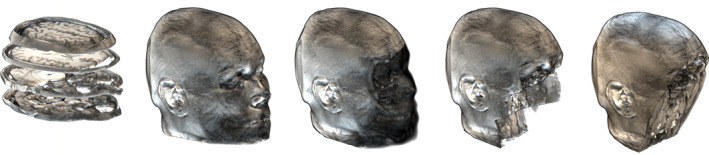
The typical structural MRI of the brain is made up of a series of 2D slices (left) from which it is easy to reconstruct a face. Pseudonymization procedures (from the middle to right) go from blurring/masking the face to zero‐out an entire part of the image, increasing anonymity but decreasing usage and sometimes damaging the frontal part of the brain. (This image was made from the MRI of one of the authors, CP, visualized with MRICRoGL, masked using mask_face (https://nrg.wustl.edu/software/face‐masking/usage/), mri_deface from the freesurfer suite (https://surfer.nmr.mgh.harvard.edu/fswiki/mri_deface) and SPM12 (https://www.fil.ion.ucl.ac.uk/spm/software/spm12/) — (https://doi.org/10.7488/ds/2877)
